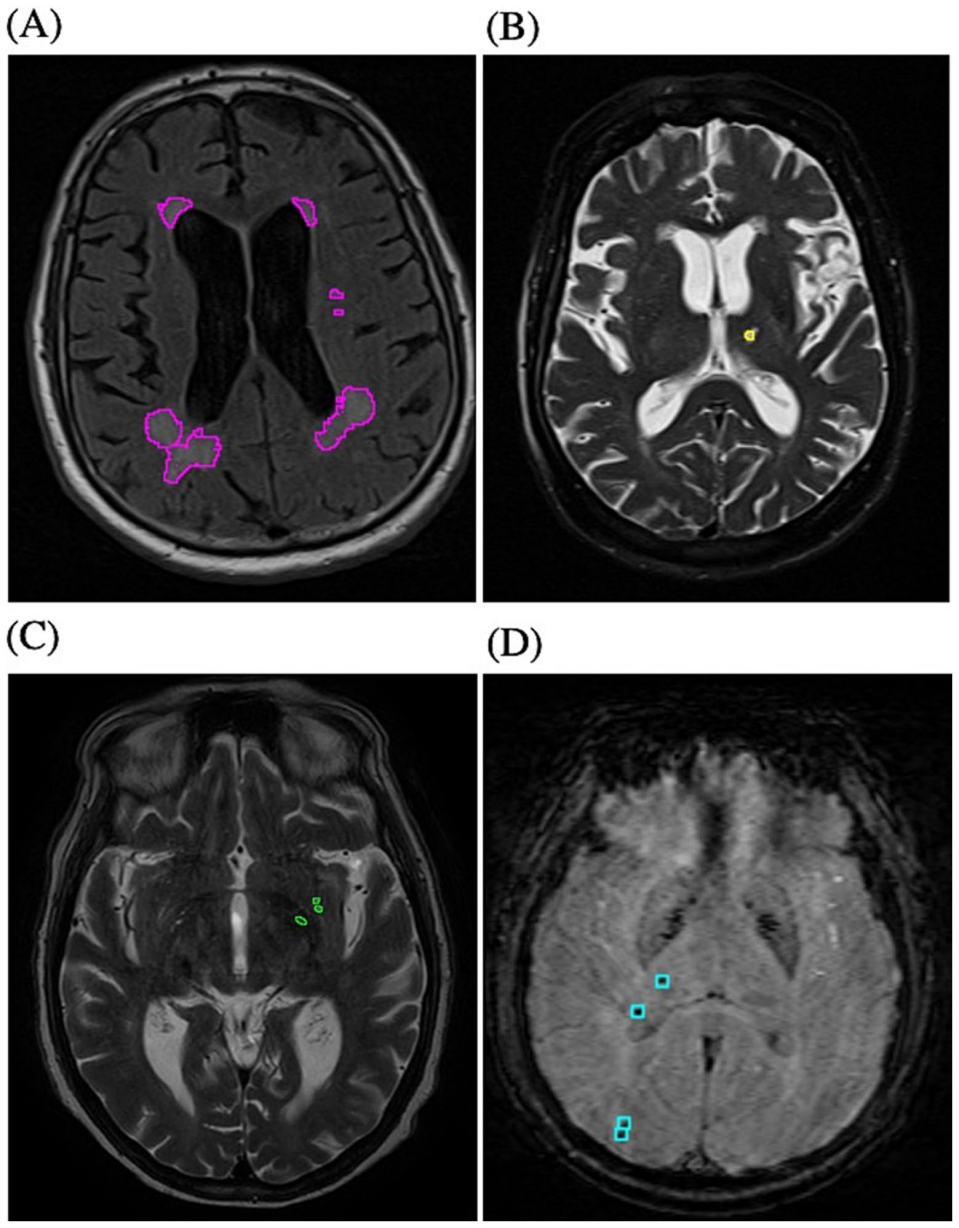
Semi-automated segmentation of SVD markers on MRI, including white matter hyperintensities seen on FLAIR (A, purple), lacunar infarcts on T2 (B, yellow), perivascular spaces on T2 (C, green), and cerebral microbleeds on SWI (D, blue).
